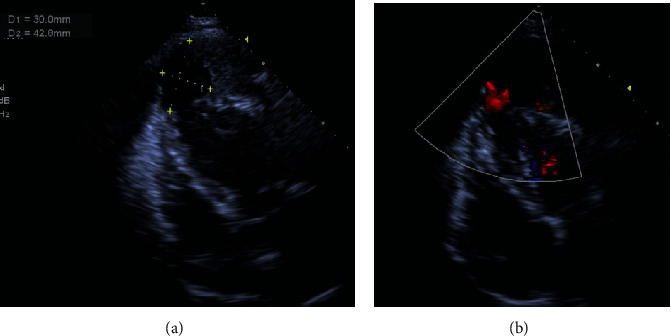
Four-chamber view TTE: the pseudoaneurysm did not change in the size (a) and active bloodstream to the pseudoaneurysm on color Doppler (b).
